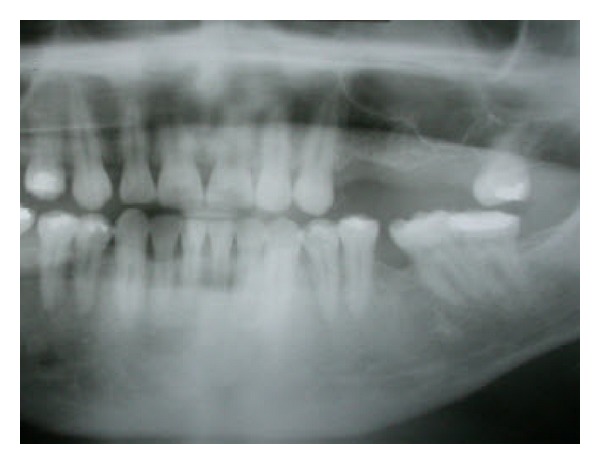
Baseline orthopantomogram showing that the subantral bone height was limited at the maxillary posterior left quadrant, with severely resorbed alveolar bone height at the site of the first maxillary molar.
